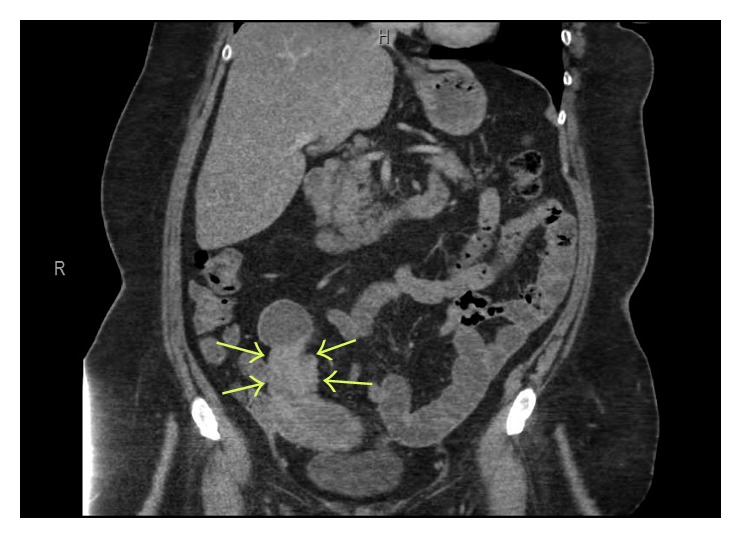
Coronal abdominal computed tomography revealing ileoileal intussusception (arrows).
